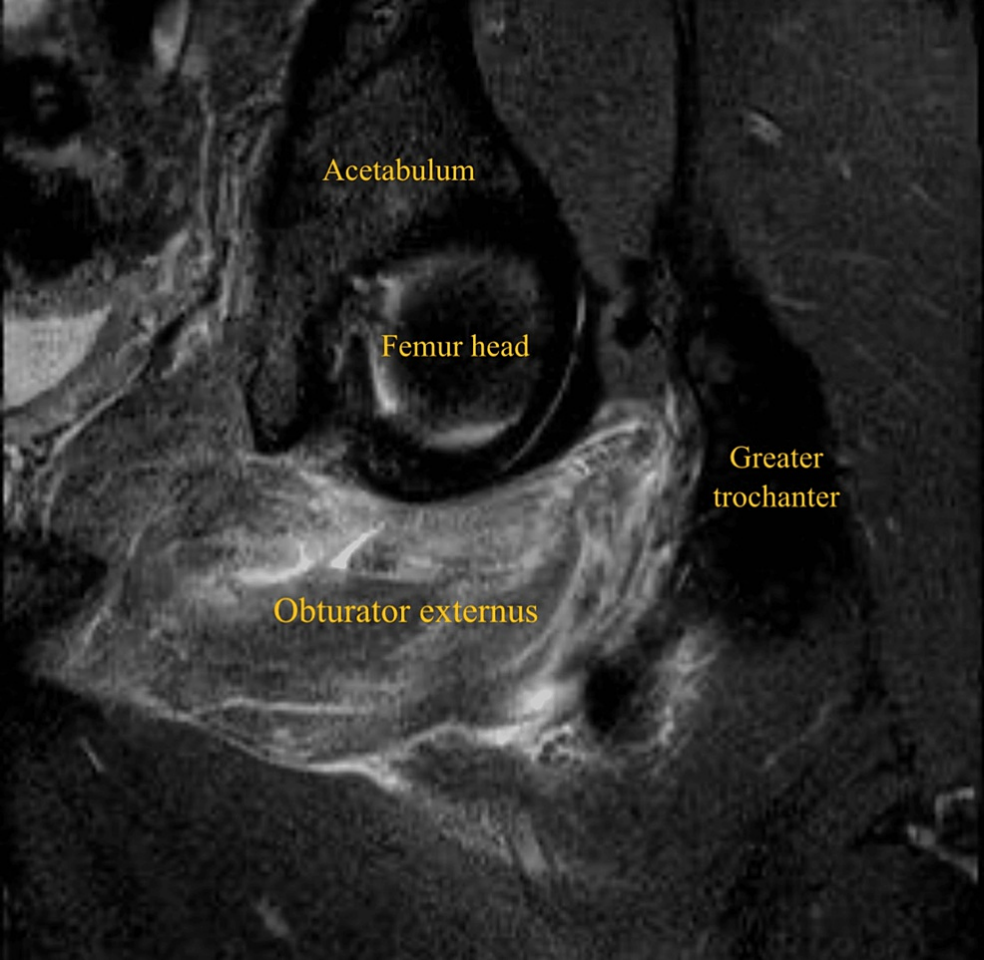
Coronal short-tau inversion recovery (STIR) MRI of the left thigh showing left obturator externus tear
